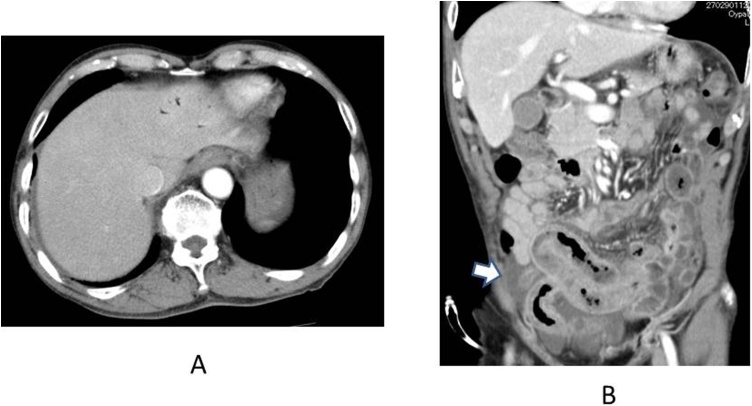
CT on the next day after the onset. Small HPVG remained at lateral segment (2A) and increased ascites (2B: indicated by white arrow). Intestinal pneumatosis was distinguished.
